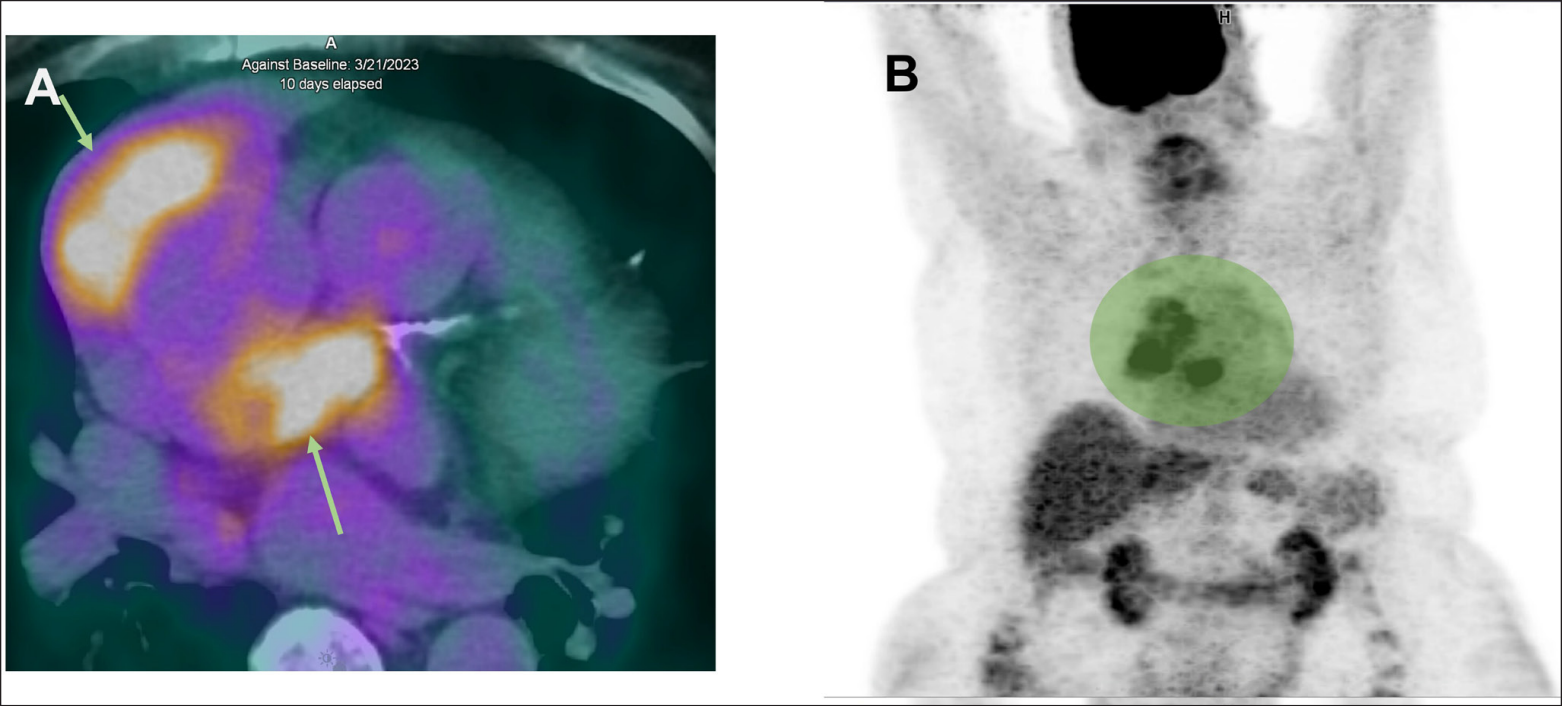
(A) Fluorine-18-fluorodeoxyglucose (18F-FDG) positron emission tomography/computed tomography shows positive FDG uptakes in periaortic pericardial masses (arrows). (B) Corresponding whole body PET/CT imaging with green area showing increased metabolic activity and FDG uptake but no evidence of mediastinal lymph node or extracardiac metastasis.
